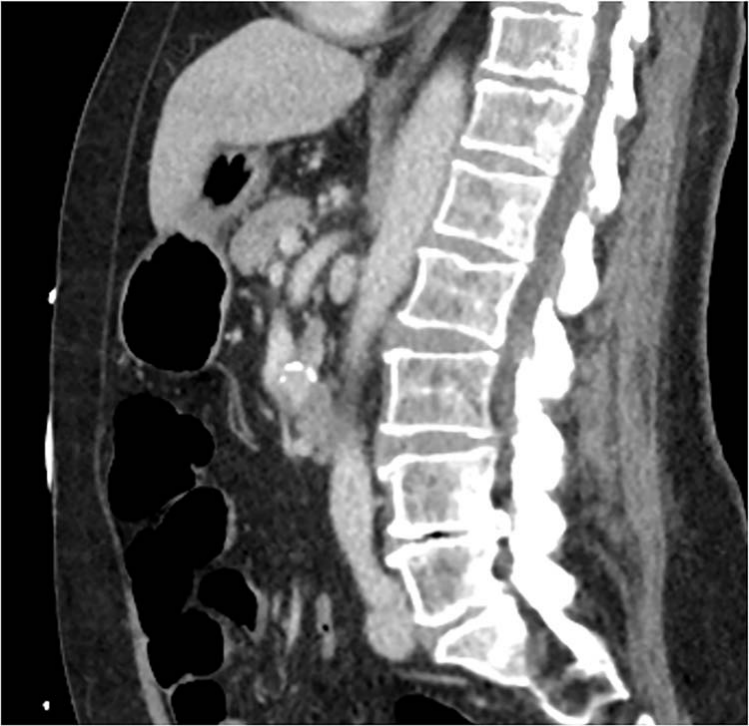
Initial CT: sagittal view of the indeterminate pancreatic/duodenal lesion.
